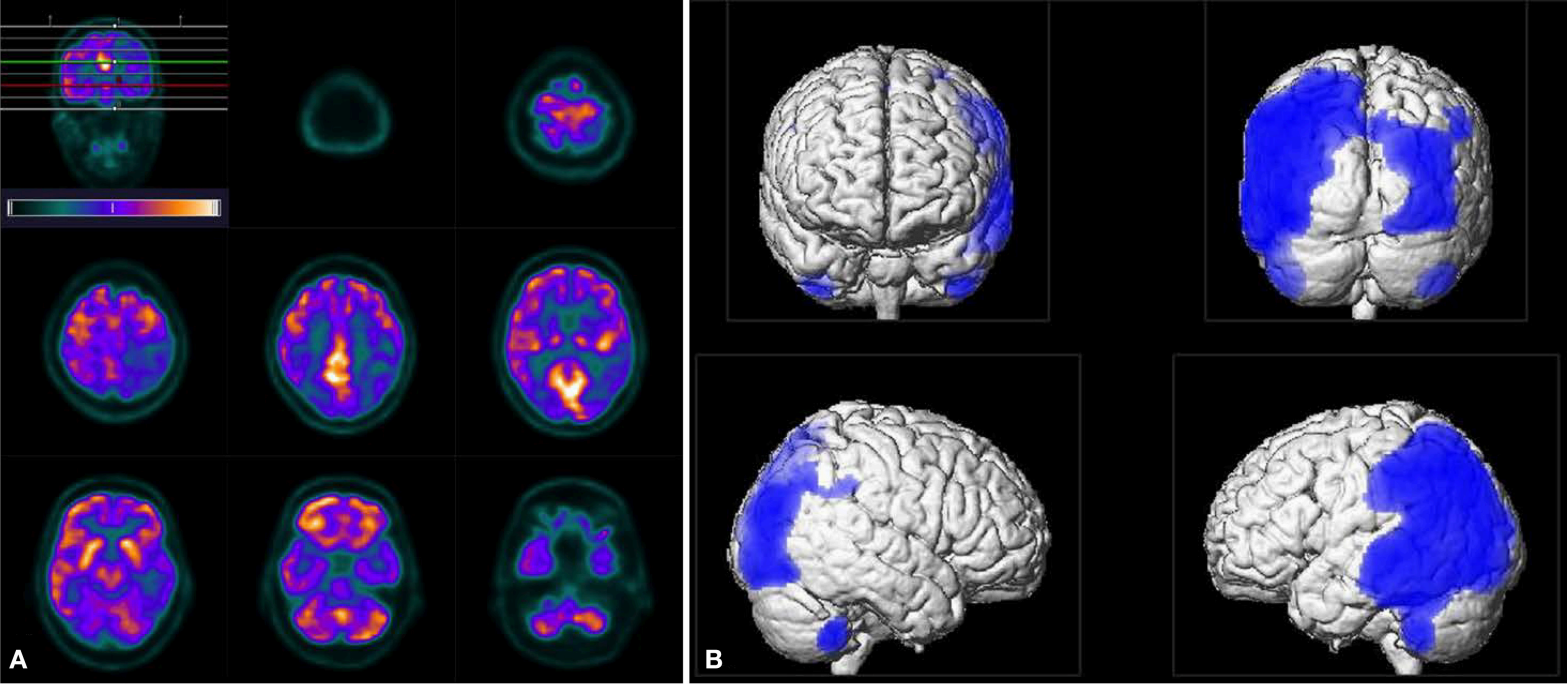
Tomograms of ^18^F-FDG uptake (A) as well as the result of SPM analysis (B, using an uncorrected *p* < 0.001 for statistical inferences) revealed distinct bilateral hypo-metabolism pronounced on the left side in parietotemporal and occipital areas. Note, unlike in tomograms the left side appears left in the SPM images.
